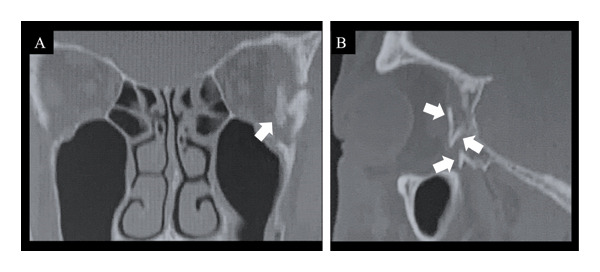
Preoperative CT image. (A) Coronal CT image. A posterior lateral orbital fracture is observed. (B) Sagittal CT image. Three bone fragments are observed posterior to the orbit. CT: computed tomography.
